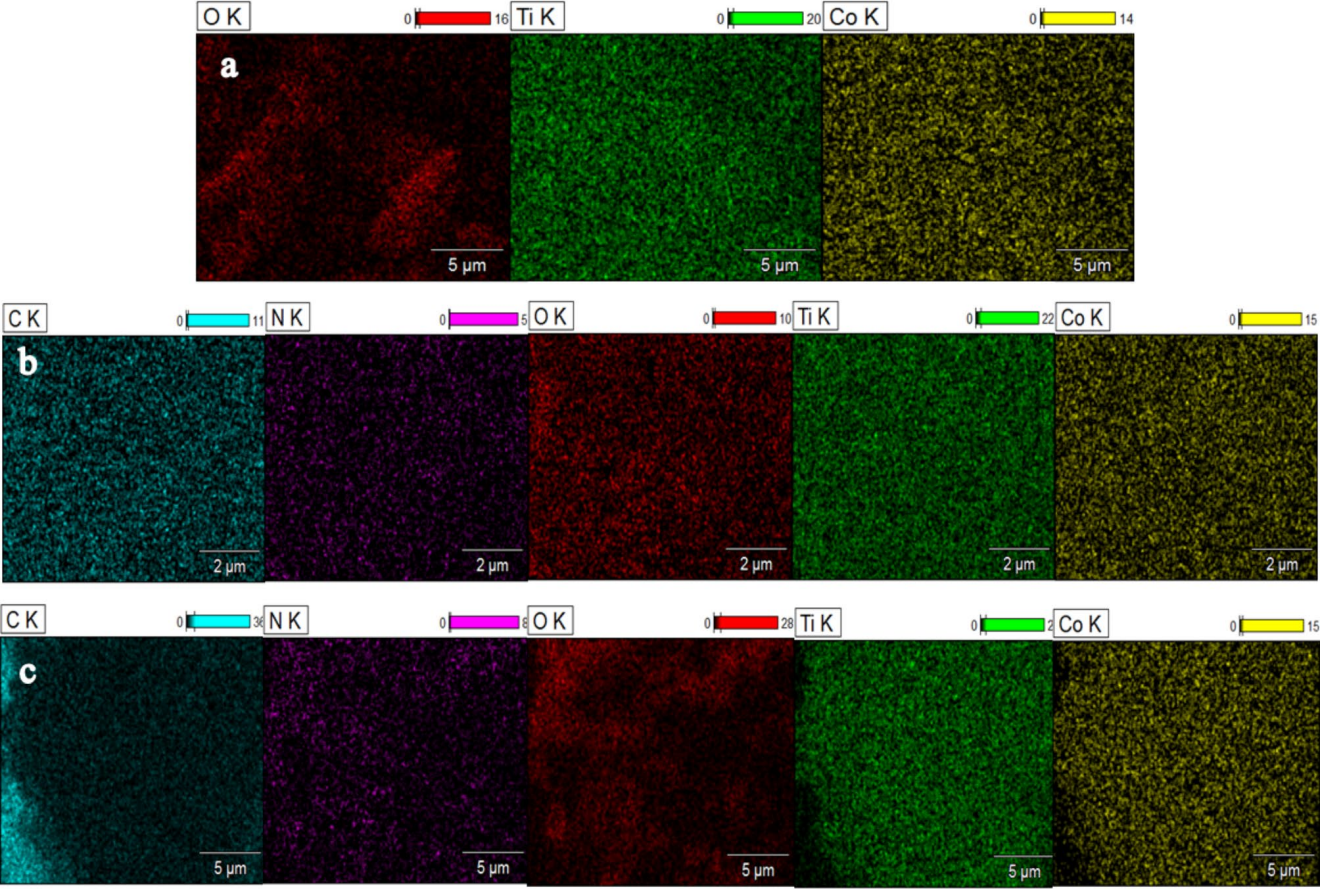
Energy dispersive X-ray mapping of: (a) CoTiO_3_, (b) 1wt.% PANI@CoTiO_3_, and (c) 2wt.% PANI@CoTiO_3_.
